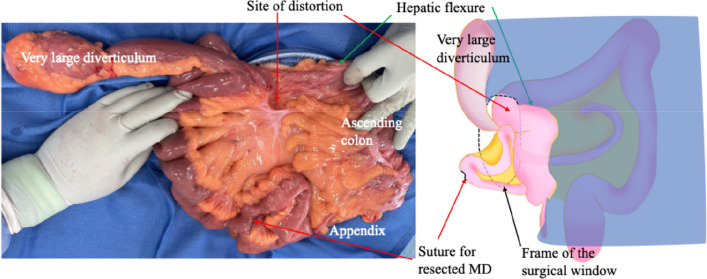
Intraoperative findings (after resecting the smaller diverticulum). A very large diverticulum was identified and removed from the peritoneal cavity. As predicted by preoperative CT, the ascending colon was volvulated anteriorly, and a considerable length of the intestinal loop was displaced laterally, passing behind the ascending colon. Before this surgical view was established, a 60-mm diverticulum located on the antimesenteric side was resected. The very large diverticulum was also located on the antimesenteric side, and its base was very close to the distorted site of the colon
